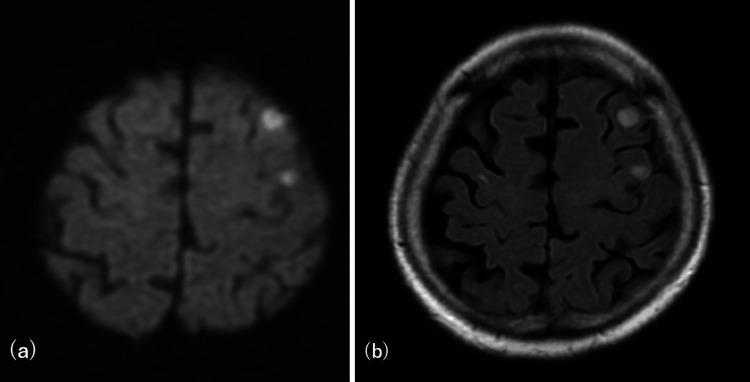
MRI two weeks prior to admission. Hyperintense lesions appeared on the left frontal lobe MRI: magnetic resonance imaging (a) Diffusion-weighted imaging (DWI). (b) Fluid attenuated inversion recovery (FLAIR)
